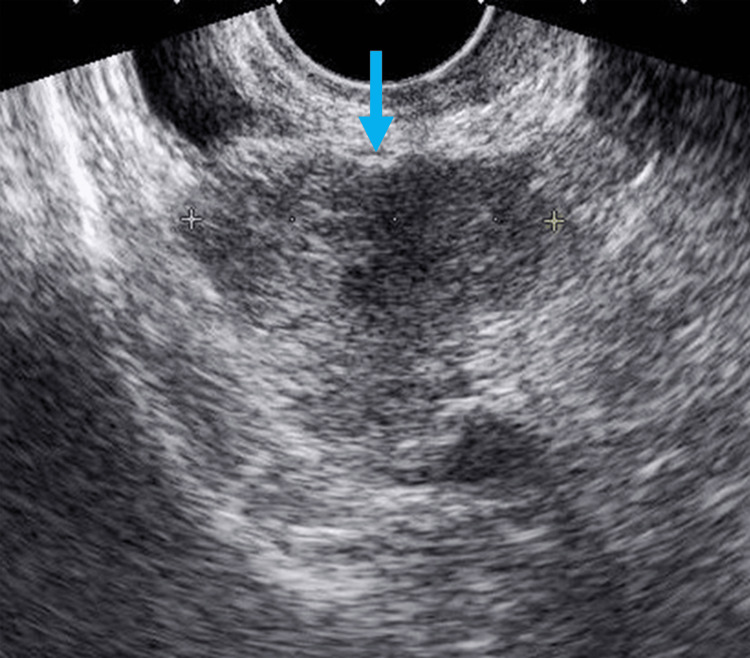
Ultrasonography findings Grayscale ultrasonography shows a hypoechoic mass on the right side of the pelvis (blue arrow).
